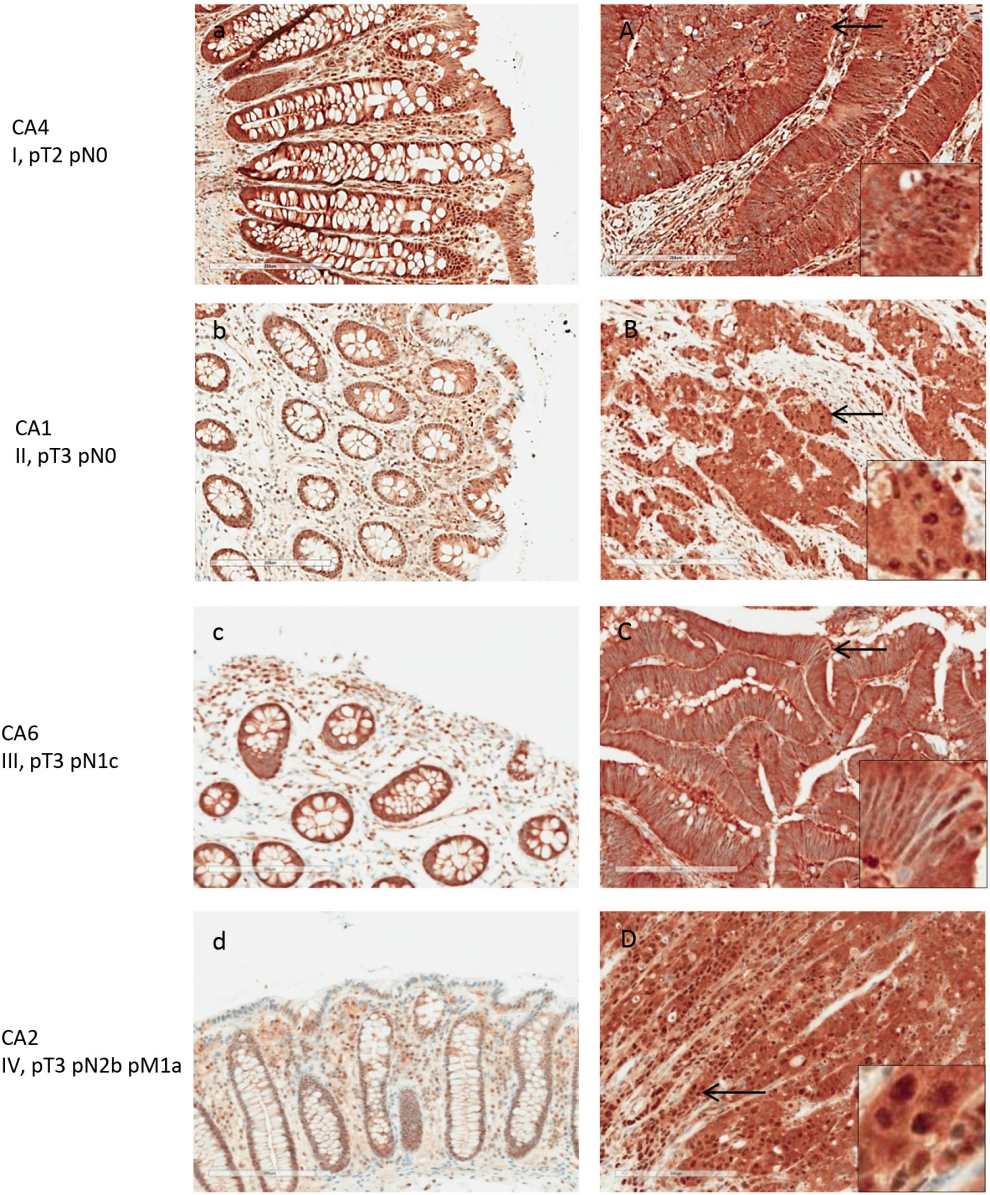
PSMA1 expression in normal and adenocarcinoma colon tissue by IHC Matched pairs of normal (a-d) and tumor (A-D) tissues from patients with stage I-V colon cancers are shown. PSA1 expression was present in normal colon tissue at moderate levels but increased significantly in cancer tissue.
